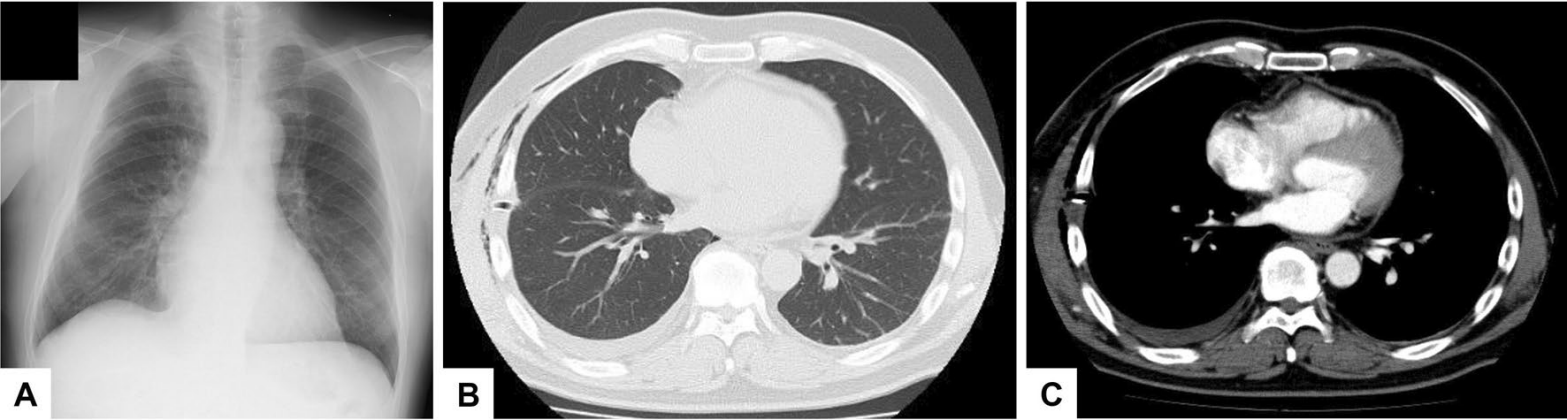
On chest radiography after surgery (A), the pneumopericardium seen preoperatively on the left side of the heart had disappeared, and the protruding right atrium on the right side of the heart had returned to the mediastinum. Computed tomography after surgery with lung window settings (B) shows that the right atrium was pushed back into the mediastinum by the inflated lung. The enhanced computed tomography after surgery with mediastinal window settings (C) shows that the right atrium was present at the site of the pericardial defect, consistent with the surgical findings
